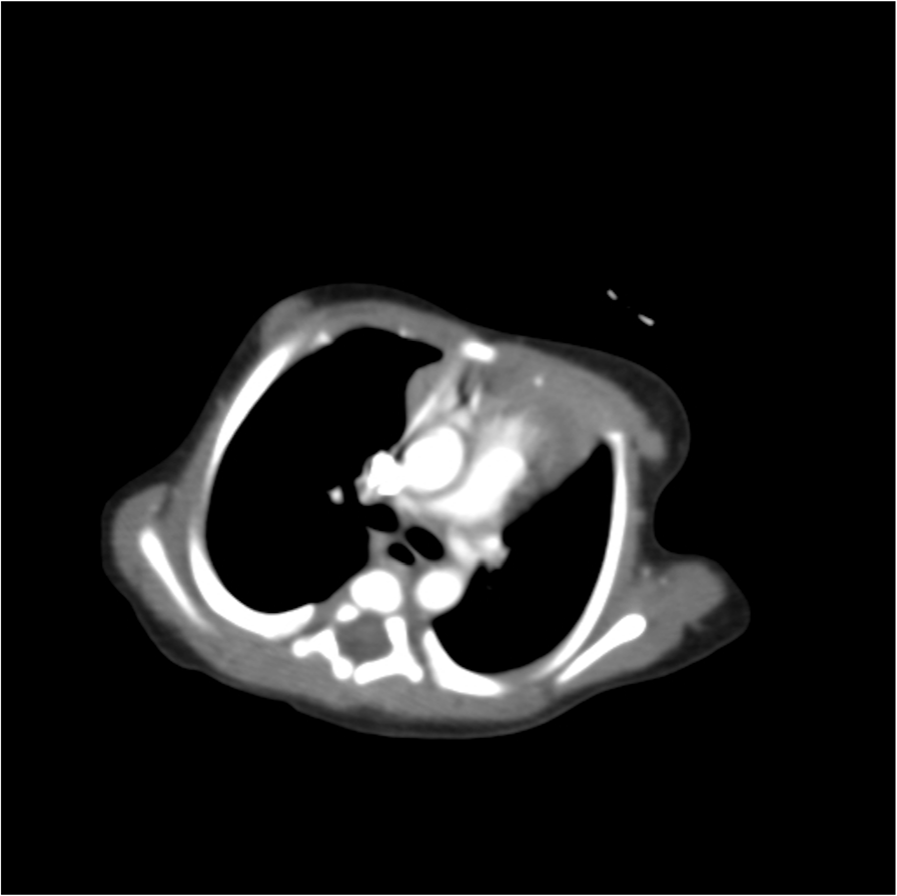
CT pulmonary angiography showing eccentric filling defect at the origin of left pulmonary artery
